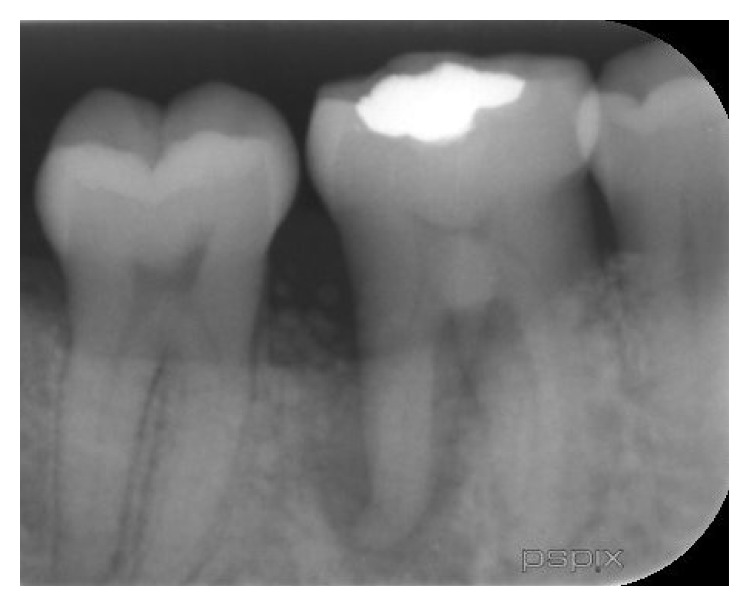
Preoperative periapical X-ray.
